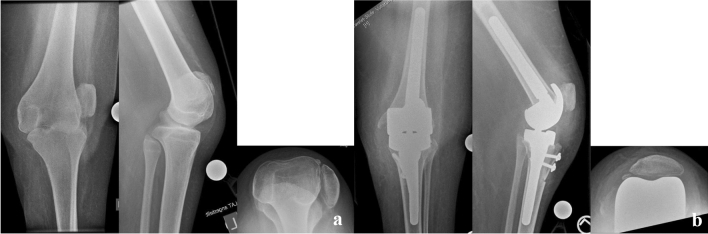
a Preoperative radiograph of the painful knee in subluxation. b Postoperative radiograph following rotating hinge knee replacement
